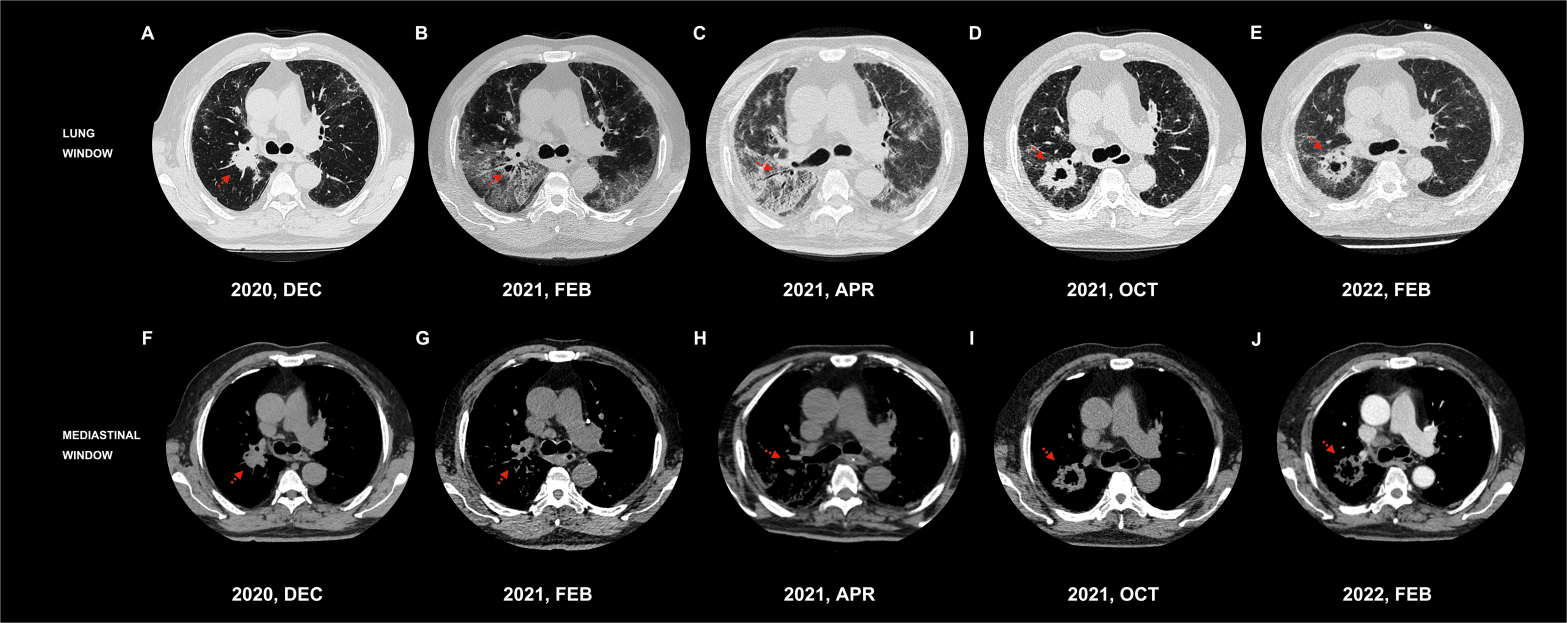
Chest CT of tracheal carina level in the lung and mediastinum windows. dynamic changes of the space-occupying lesions. (A)/(F) A space-occupying lesion (2.9 × 2.6 cm) in the upper lobe of the right lung. (B)/(G) The primary foci shrank significantly and was surrounded by GGOs and consolidation shadow. (C)/(H) Multiple GGOs, reticulation, grid lesions, and traction bronchiectasis in the dominant lobe around the primary foci with patchy lesions in the opposite lobe. (D)/(I) A recurred lobulated mass (5.0×2.6 cm) in the upper lobe of the right lung. (E)/(J) Mildly reduced lesion (4.2×4.3 cm).
